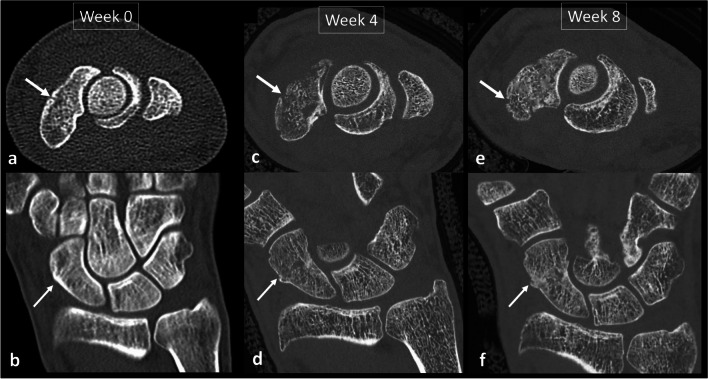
Improved spatial resolution between an EID-DECT (a, b) and clinical PCD-CT (c–f). A 47-year-old male with suspected scaphoid fracture (white arrows). The fracture was initially missed but retrospectively visible with low dose EID-CT of the wrist (CTDIvol_32_ 1.16 mGy) (a, b). Four weeks after suspected fracture (c, d), a PCD-CT was performed and demonstrated the fracture and the osteolytic changes as part of the repair process (CTDIvol_32_ 4.28 mGy). Note the higher resolution of PCD-CT with finer depiction of trabecular bone compared to DECT. PCD-CT 8 weeks after fracture (e, f) demonstrated a partly healed/immature bridging (CTDIvol_32_ 4.49 mGy)
